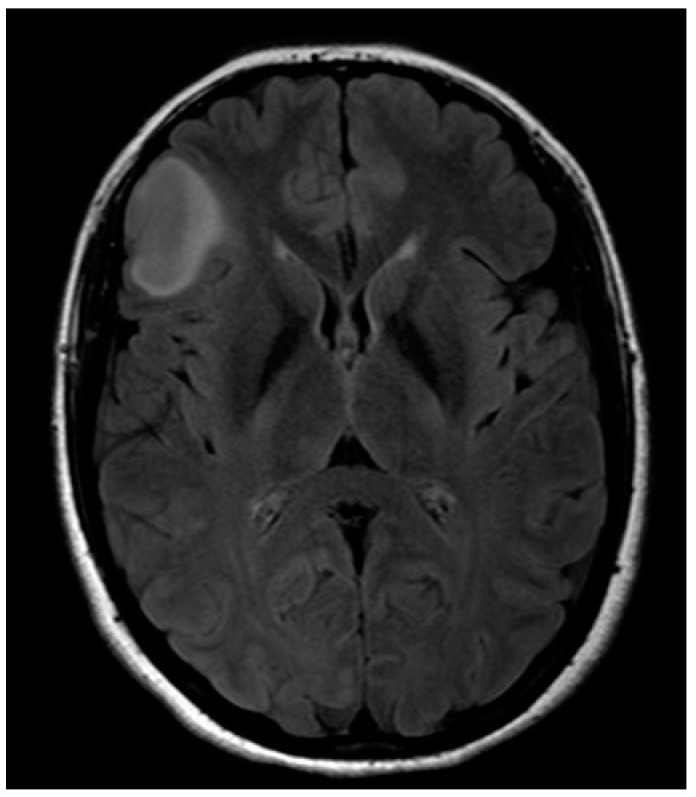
Anatomical MRI scan showing right fronto-opercular diffuse low-grade glioma.
